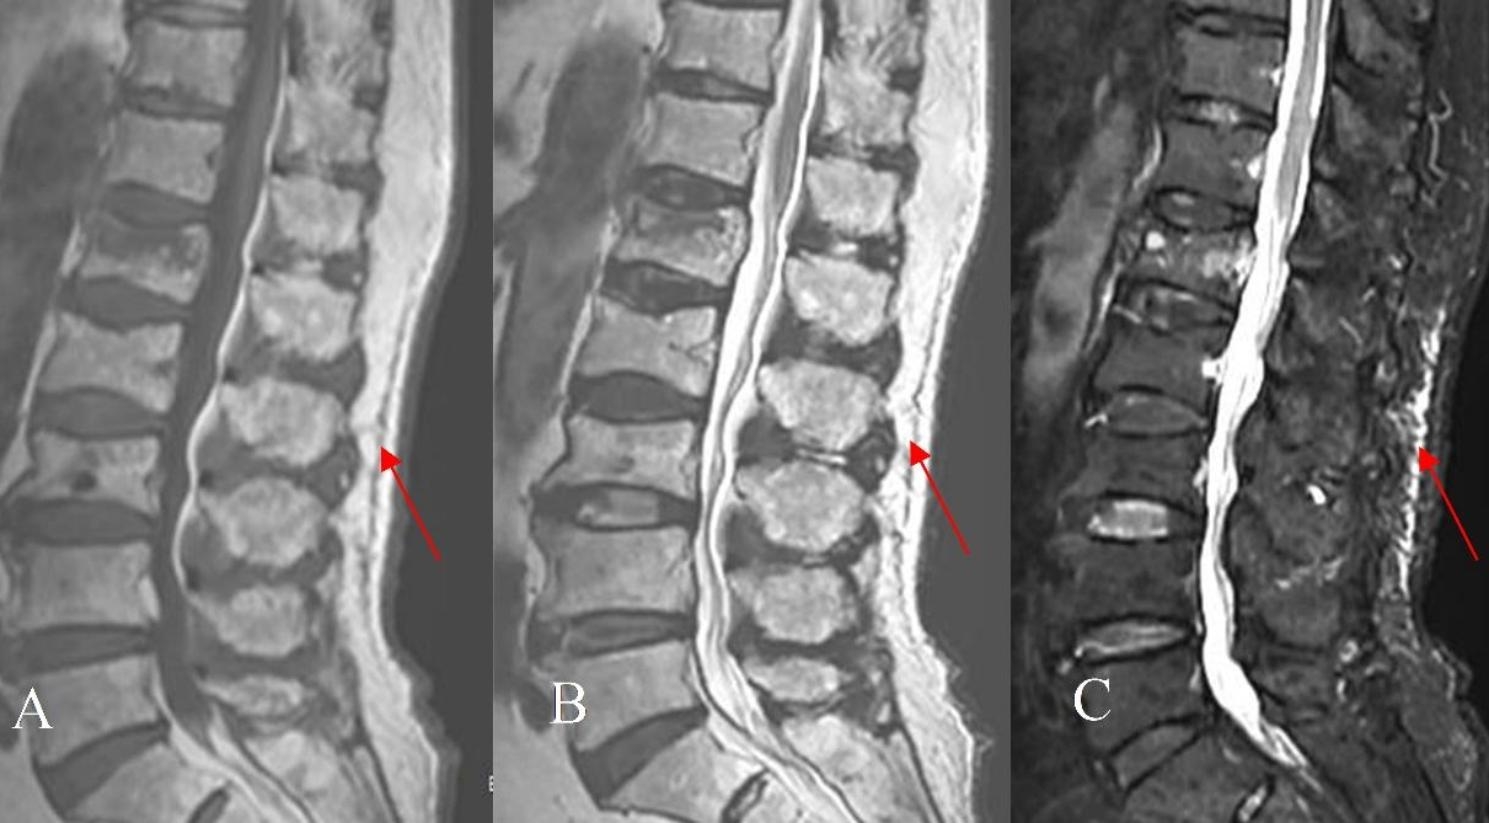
OVCF in the L1 vertebrae with TFI (Red arrows). MRI: the T1- weighted image (A) shows low signal intensity, and the T2- weighted image (B) and T2-weighted fat suppression image (C) show high signal intensity
